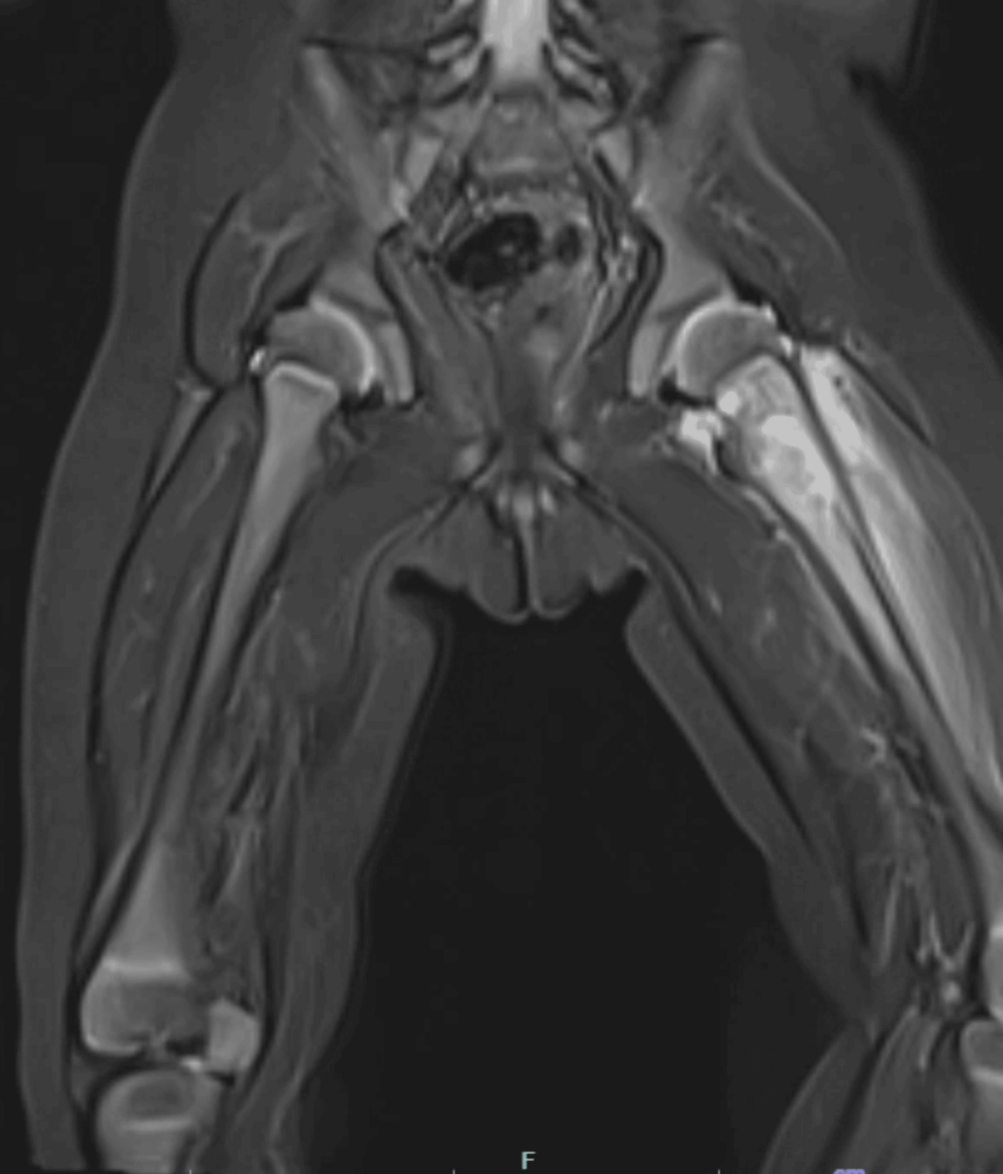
MRI of the pelvis including the midshaft of both femurs MRI of the left femur showing an ill-defined lesion in the proximal metaphysis with bone marrow edema, a subperiosteal fluid collection consistent with abscess, and extensive edema in the adjacent thigh muscles. No discrete soft tissue mass was observed apart from the fluid collection.
